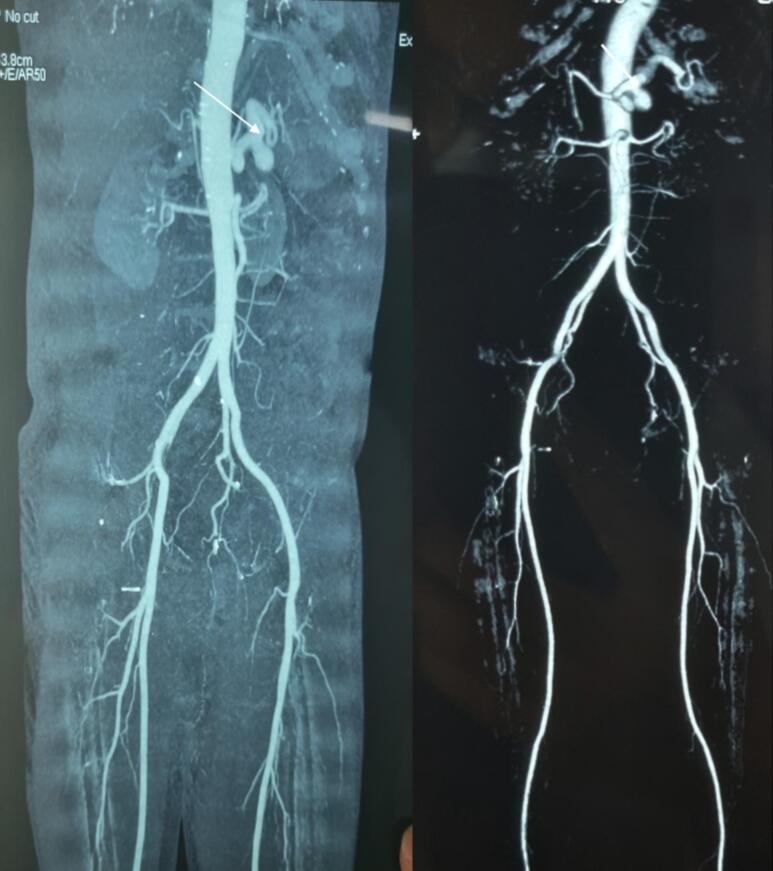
CT-angiography and 3D-reconstruction showing splenic artery aneurysm.
